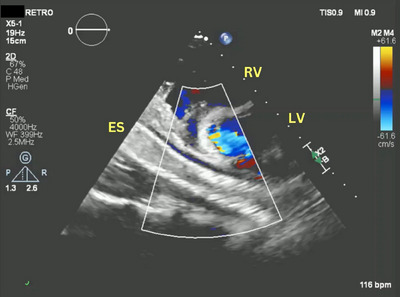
Two‐dimensional transthoracic echocardiography. Off axis short axis view. The ES is visualized in long axis posterior to LV and right RV. ES, esophageal stent; LV, left ventricle; RV, right ventricle.
